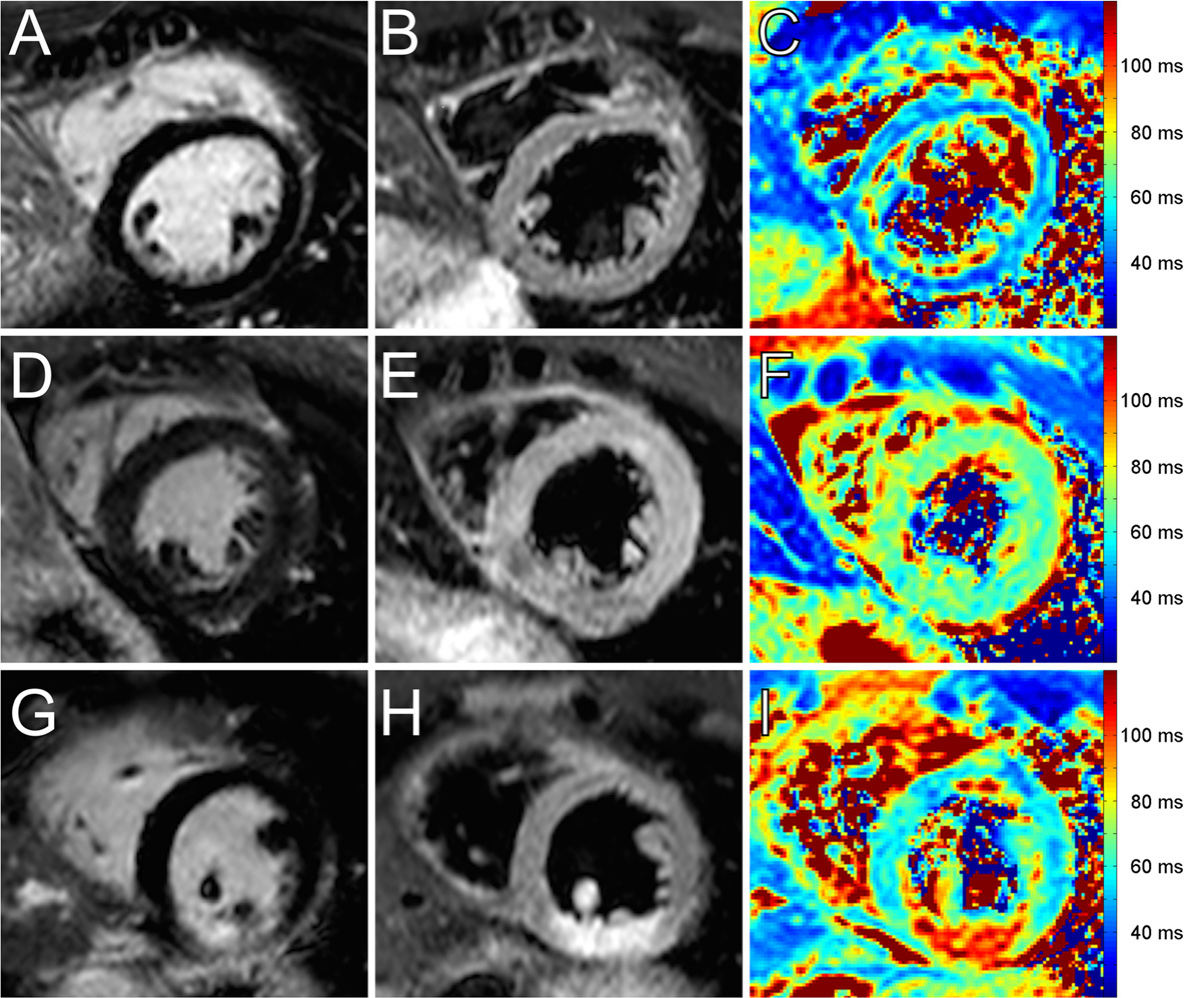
Example CMR findings. Midventricular short axis T2-weighted short tau inversion recovery (STIR), late gadolinium enhancement (LGE), and T2 mapping sequences in a 29-year-old healthy male volunteer (A, B, C), a 42-year-old patient (#1) with acute, diffuse myocarditis (D, E, F), and a 53-year-old patient (#2) with acute myocardial infarction (G, H, I). Mean global T2 relaxation time for the healthy volunteer was 48.5 ± 4.6 ms (anterior: 47.8 ms; anteroseptal: 57.8 ms; inferoseptal: 52.1 ms; inferior: 49.6 ms; inferolateral: 45.5 ms; anterolateral: 47.1 ms) (C). In patient #1 diffuse myocardial edema was present throughout all myocardial segments (D, E, F), associated with increased global T2 values of 68.0 ± 2.4 ms (anterior: 65.4 ms; anteroseptal: 68.4 ms; inferoseptal: 70.5 ms; inferior: 65.8 ms; inferolateral: 64.4 ms; anterolateral: 66.1 ms). Patient #2 showed an acute myocardial infarction of the inferior segments with subendocardial LGE (G) and pronounced edema in the T2 STIR image (H). Pronounced myocardial edema is also visible in the corresponding T2 map (I), where the infarcted segments showed mean T2 relaxation times of 89.4 ms (inferior) and 82.3 ms (inferolateral) (anterior: 55.5 ms; anteroseptal: 56.2 ms; inferoseptal: 70.5 ms; anterolateral: 66.1 ms; global T2 relaxation time: 62.5 ± 13.1 ms).
